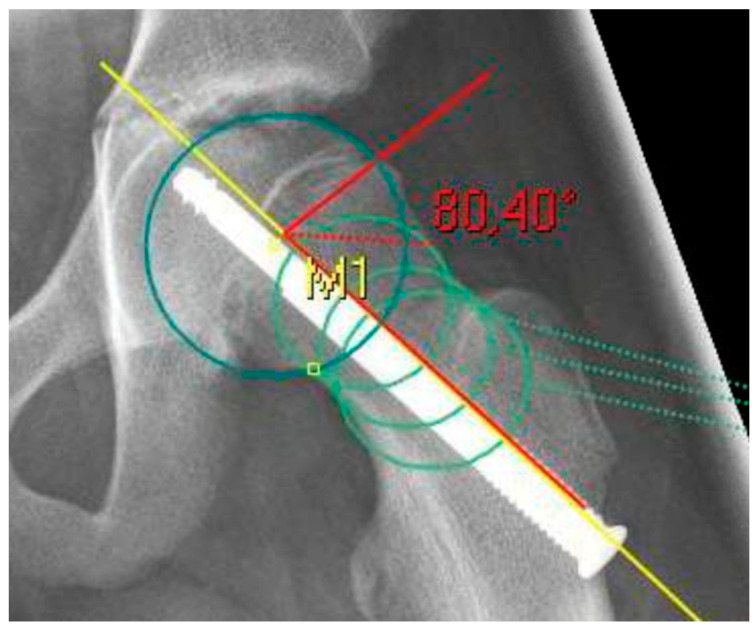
X-ray after screw fixation of a left sided SCFE as an example for the measurement of the alpha angle. The femoral neck axis (yellow line) was determined with three circles (light green) according to Bouma [29].
